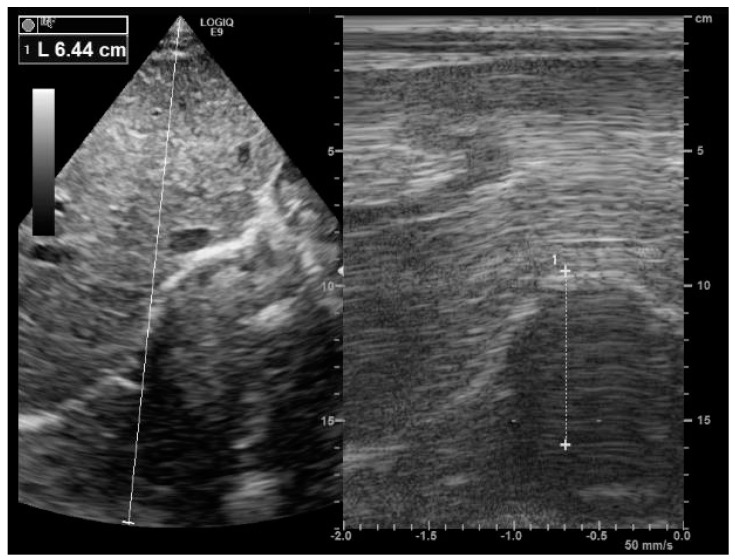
Right diaphragm ultrasound. Note the normal inspiratory motion (64 mm) of the hemidiaphragm from the subcostal view. After the visualization of the right hemidiaphragm (bright line) using a B-mode (image on the left), an M mode was applied (image on the right) to record diaphragm motion.
